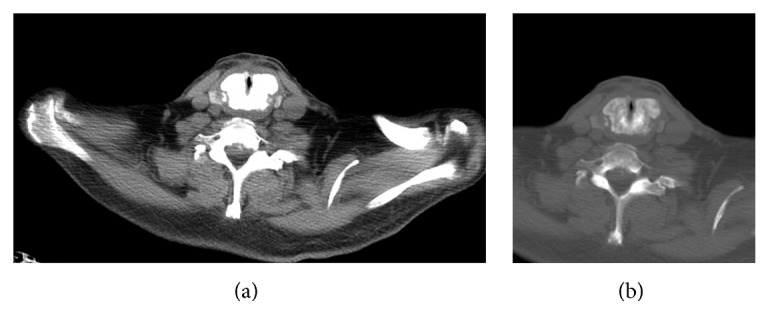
CT scan with axial sections showing enlargement and generalized sclerosis of the cricoid cartilage. Bone window where cricoid blast transformation becomes more evident.
